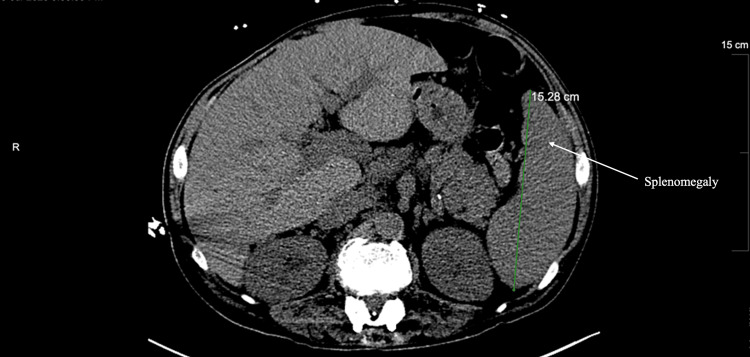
Non-contrast CT of the abdomen and pelvis upon re-presentation. Non-contrast CT of the abdomen and pelvis upon re-presentation demonstrating hepatomegaly, gallbladder decompressed with cholecystostomy tube, and an enlarged spleen measuring 15 cm.
